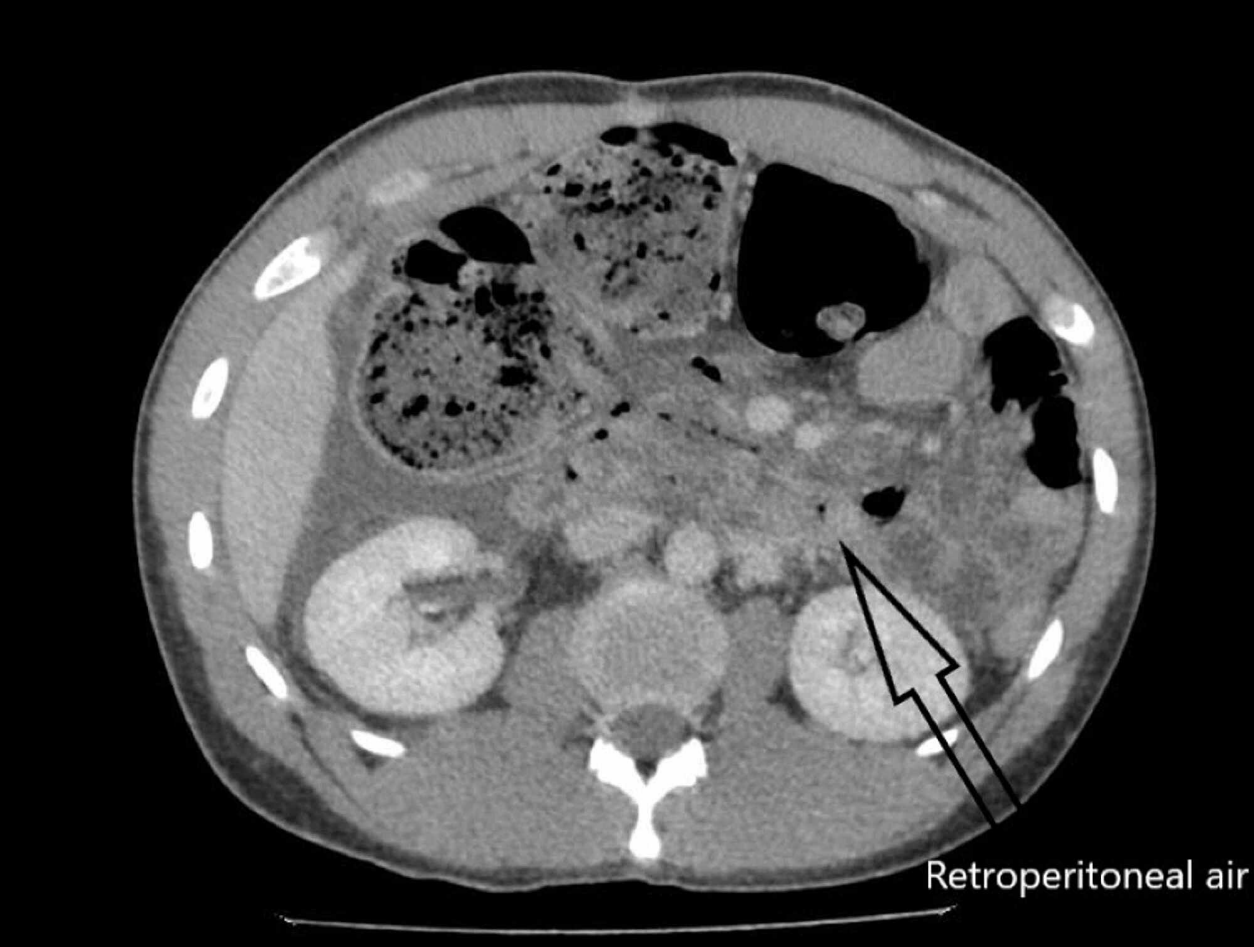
Axial section of CT showing retroperitoneal air
